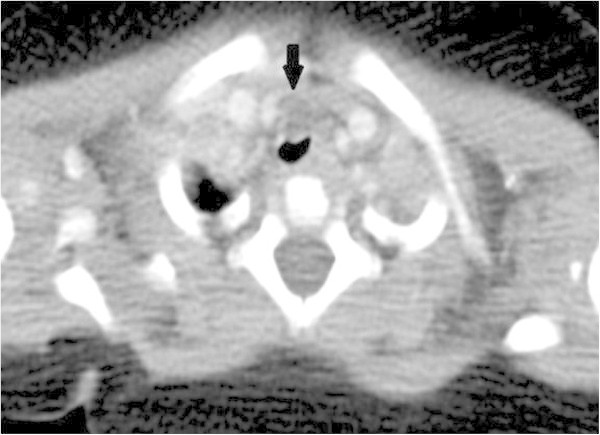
CT findings: a cystic mass compressing the anterior wall of the trachea (Arrow).
